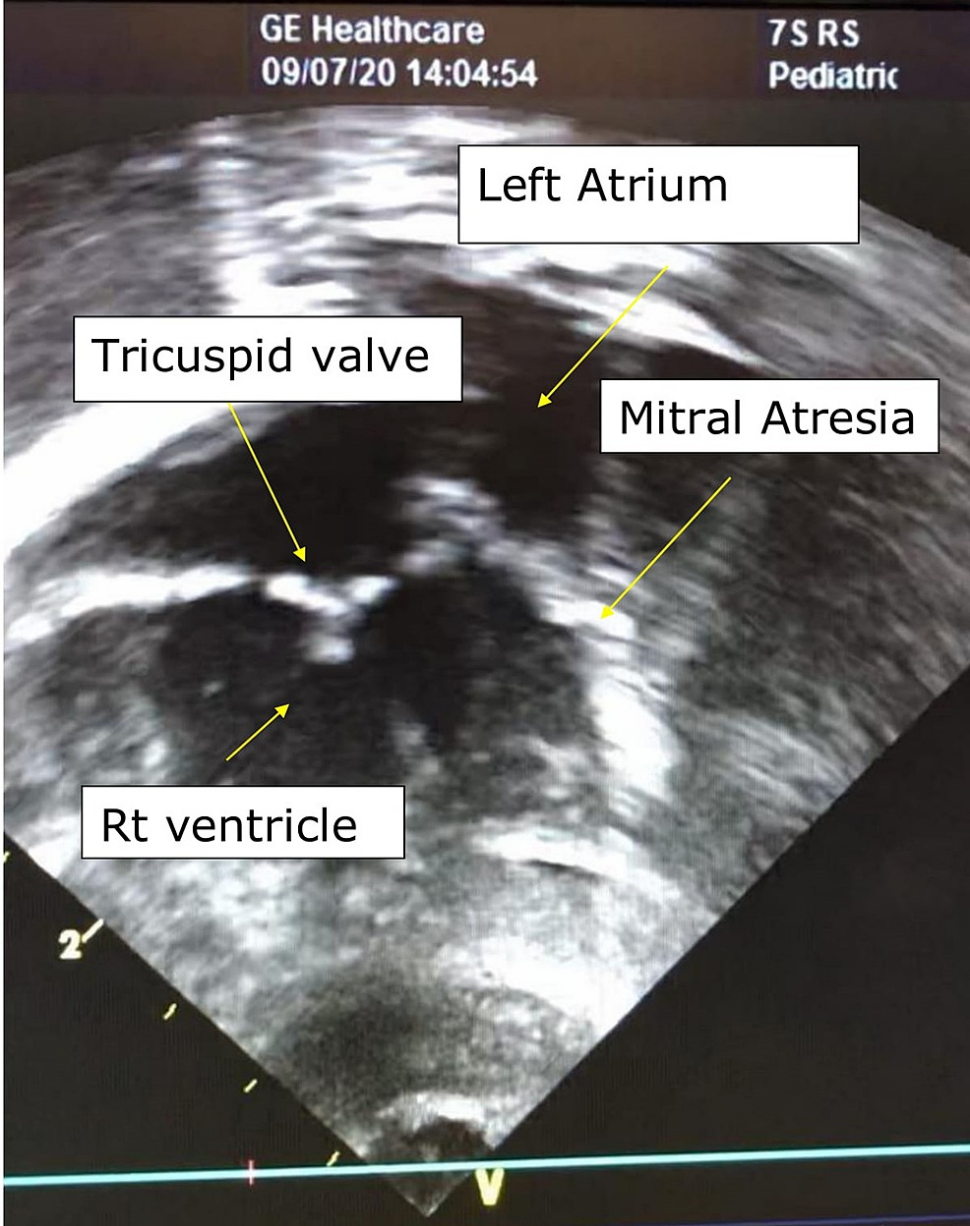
Echocardiography findings - apical four-chamber view shows atretic mitral valve and severe hypoplastic left ventricle
